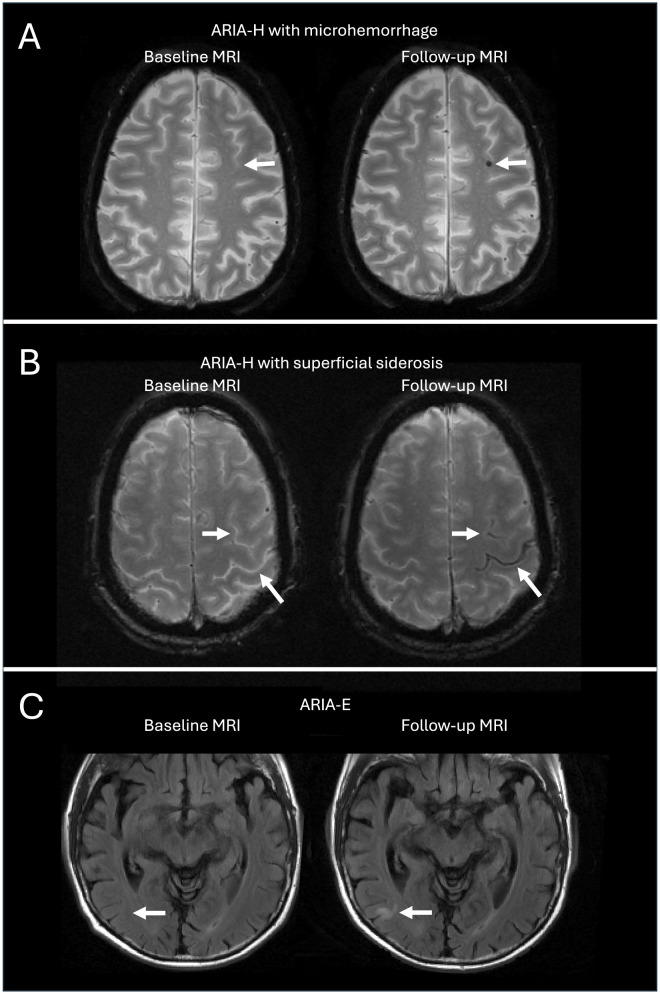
Representative images of ARIA in the A4 study. (A) ARIA-H with microhemorrhage appears as small parenchymal areas of signal void with associated blooming artifact on T2-star sequence. (B) ARIA-H with superficial siderosis is visible as hypointense signal in the leptomeninges on T2-star sequence. (C) ARIA-E refers to parenchymal edema manifesting as hyperintensities on T2-weighted FLAIR sequence. Axial T2-star (A, B) and FLAIR (C) images of the brain obtained before (left, “Baseline MRI”) and after (right, “Follow-up MRI”) the development of ARIA. In each panel, white arrows indicate the same cerebral area in the two MRI scans.
